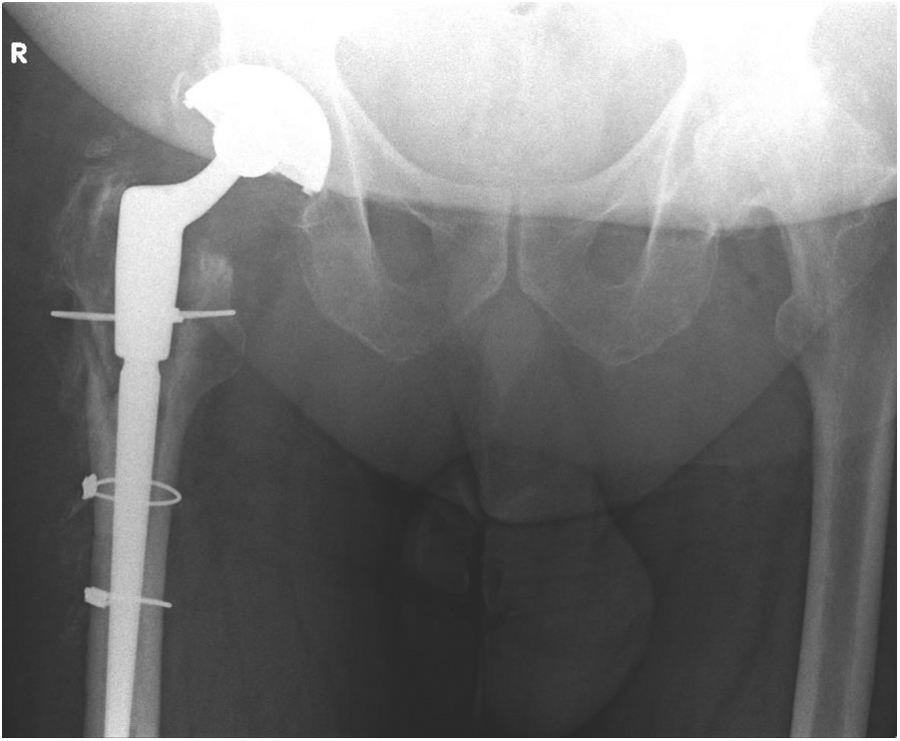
Postoperative X-ray after extended trochanteric osteotomy (ETO) and restoration stem insertion.
